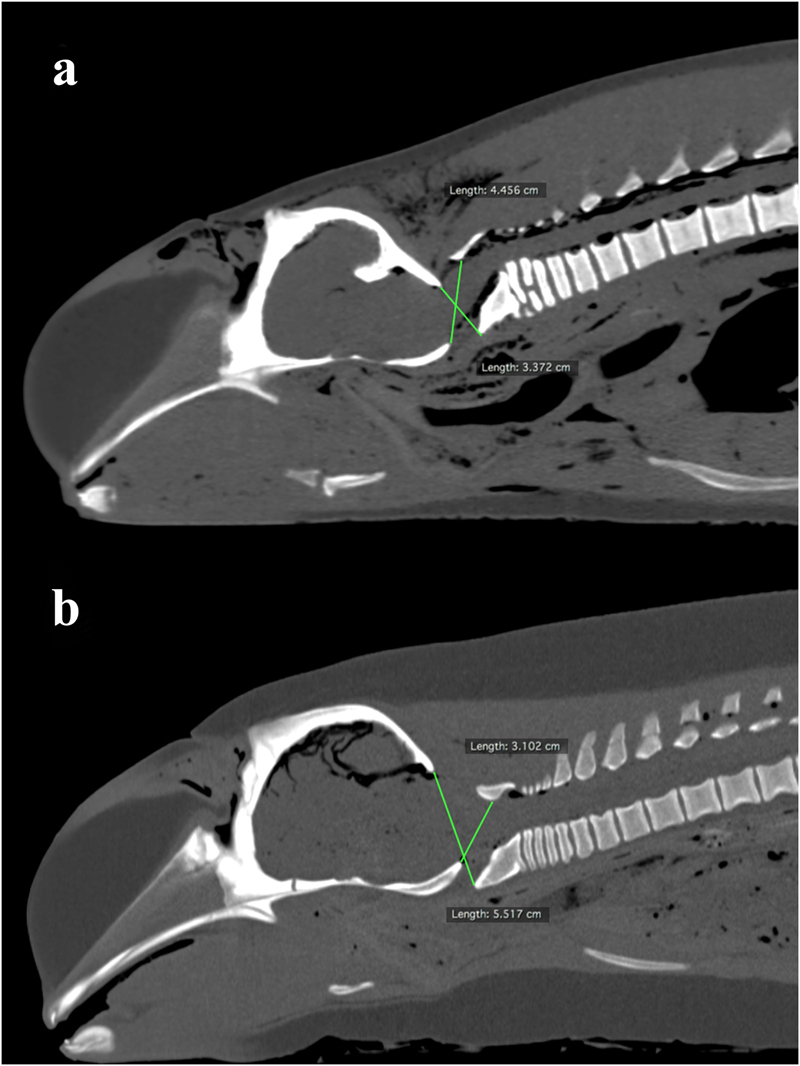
Reconstructed midsagittal postmortem computed tomography images of finless porpoise diagnosed with atlanto-occipital dissociation (AOD) in dorsal-ventral dimension, (a) dorsally displaced AOD and (b) ventrally displaced AOD.
